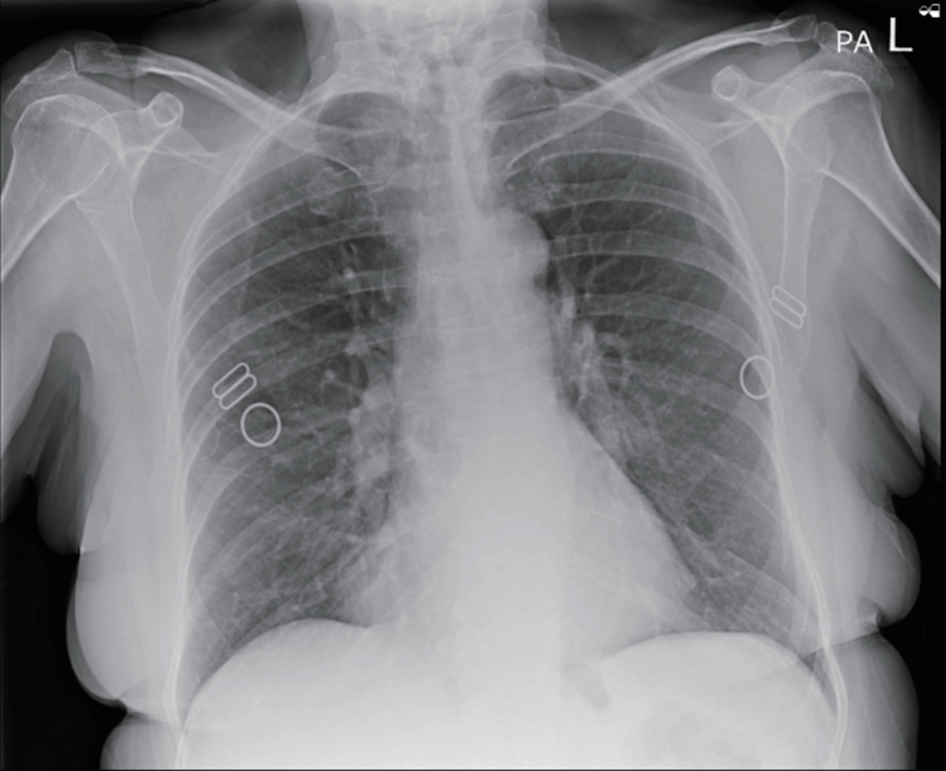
Anterior-posterior chest X-ray, 3 years prior to presentation showing normal chest X-ray and no gas loops in the hemidiaphragm.
